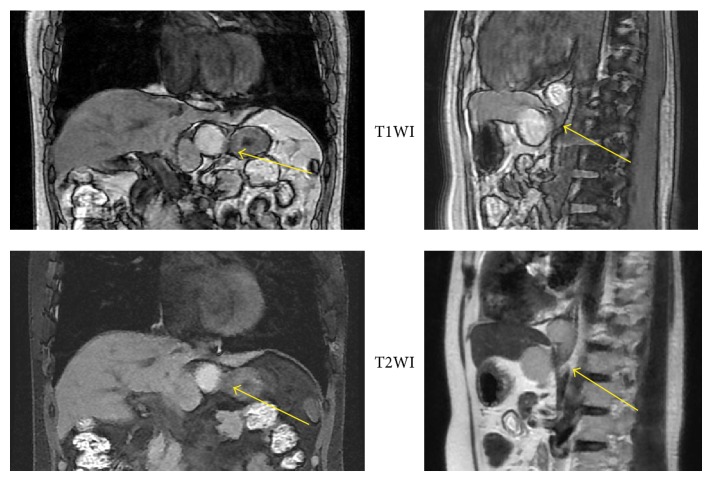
Magnetic resonance imaging. The images reveal a cystic mass extending from the lower esophagus to the cardiac region of the stomach. The mass appears to be located in or even outside the muscular layer and may contain mucin, high protein fluid, or blood.
